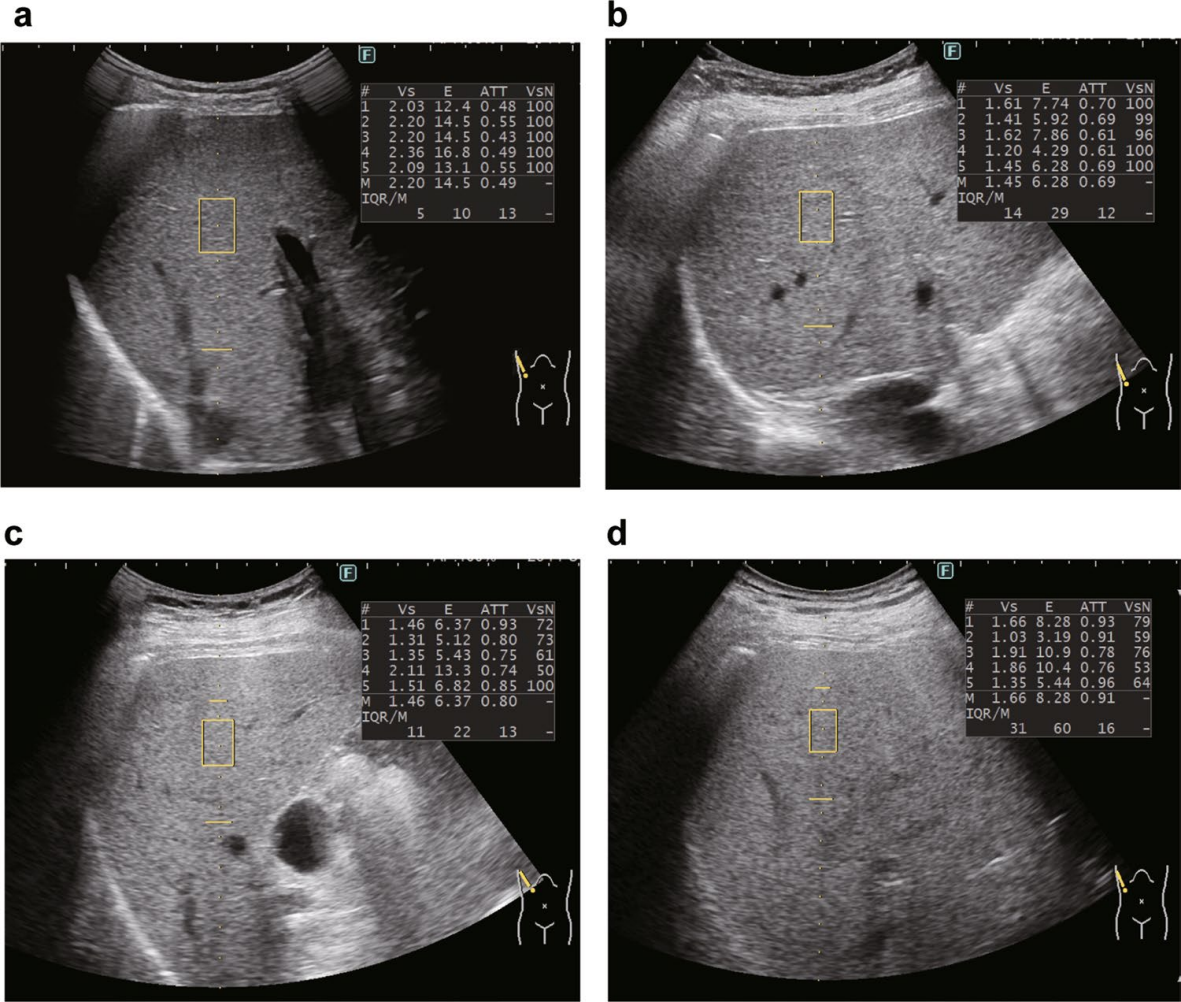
Measurement of attenuation coefficient with iATT. Ultrasound images are presented for each S grade defined by MRI-PDFF. a Steatosis grade 0 (iATT value, 0.49; IQR/M, 13%; minimum value of VsN, 100), b steatosis grade 1 (iATT value, 0.69; IQR/M, 12%; minimum value of VsN, 96), c steatosis grade 2 (iATT value, 0.80; IQR/M, 13%; minimum value of VsN, 50), d steatosis grade 3 (iATT value, 0.91; IQR/M, 16%; minimum value of VsN, 53). *IQR/M* interquartile range/median, *MRI-PDFF* magnetic resonance imaging-proton density fat fraction
